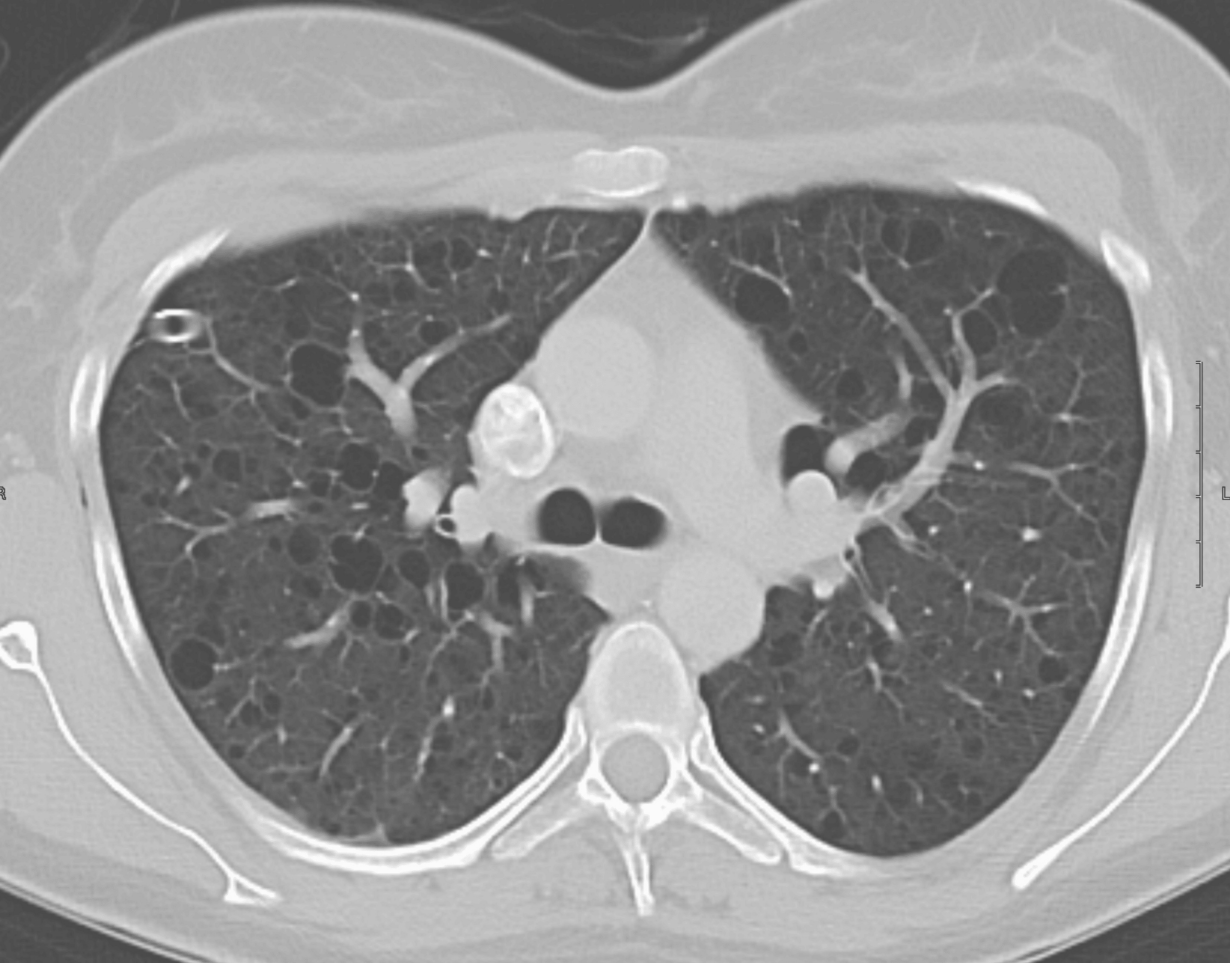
Contrast-enhanced CT of the upper lung zones showing numerous bilateral discrete cystic spaces with very thin or imperceptible walls Lung volumes are normal with no evidence of emphysema CT: computed tomography
